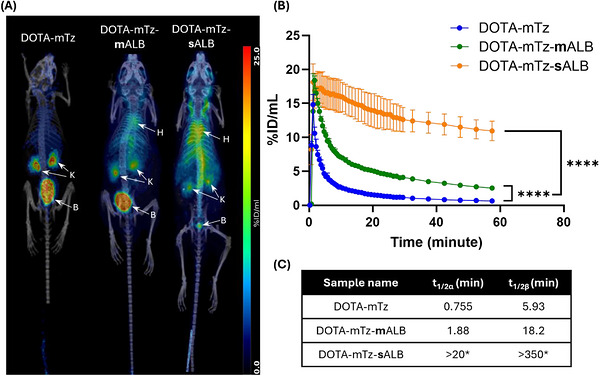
Pharmacokinetic study of clickable albumin binding radioligands. (A) In vivo biodistribution of radioligands captured by dynamic PET‐CT scans within a 7–11 min interval post‐injection (H: heart; K: kidney; B: bladder) (Left: [^64^Cu]Cu‐DOTA‐mTz; Middle: [^64^Cu]Cu‐DOTA‐mTz‐mALB; Right: [^64^Cu]Cu‐DOTA‐mTz‐sALB); (H: heart; K: kidney; B: bladder); (B) Time‐activity curves (TAC) of in vivo cardiac signal comparison within the first 60‐min post‐injection (the values are expressed as mean ± SD, *n* = 4); (C) Half‐life estimation derived from biexponential fitting of cardiac signals. t_1/2α_: distribution half‐life; t_1/2β_: elimination half‐life. *For [^64^Cu]Cu‐DOTA‐mTz‐sALB, these values are presented only as descriptive estimates and interpreted cautiously, which were determined by a cardiac TAC up to 48 h post‐injection (Figure S21). Statistical significance was justified using one‐way ANOVA with Dunnett's multiple comparison tests, the asterisks denote ^^
